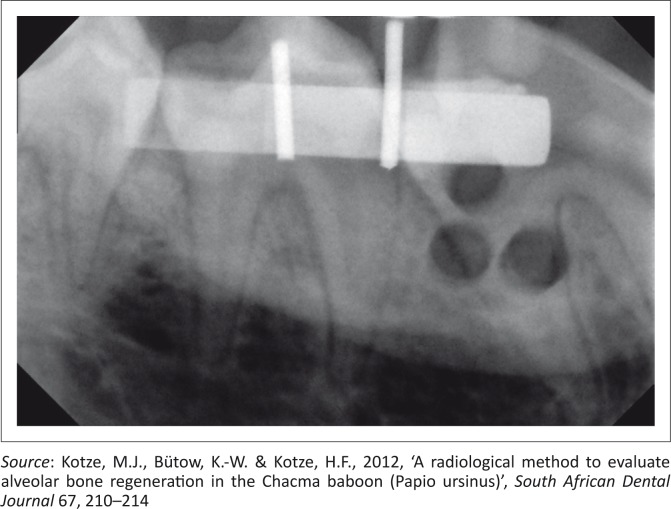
Directly post-operatively radiograph of right mandible with the three bony defects visible.
